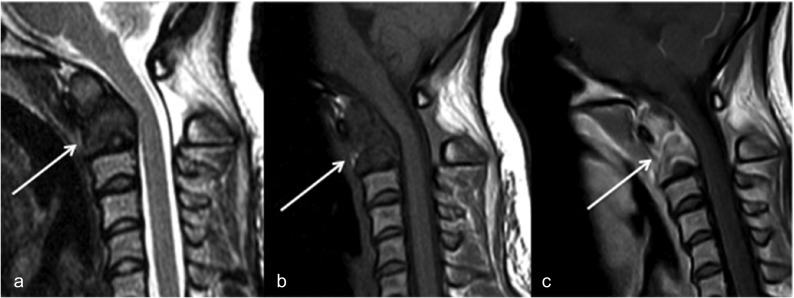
T2-weighted, T1-weighted, T1-weighted with intra-venous contrast administration midline sagittal MR images of the cervical spine showing the low signal intensity of the C2 vertebral body and odontoid process (a, b), with enhancement after contrast administration (c), and a solution at the junction between the odontoid process and vertebral body (a–c).
